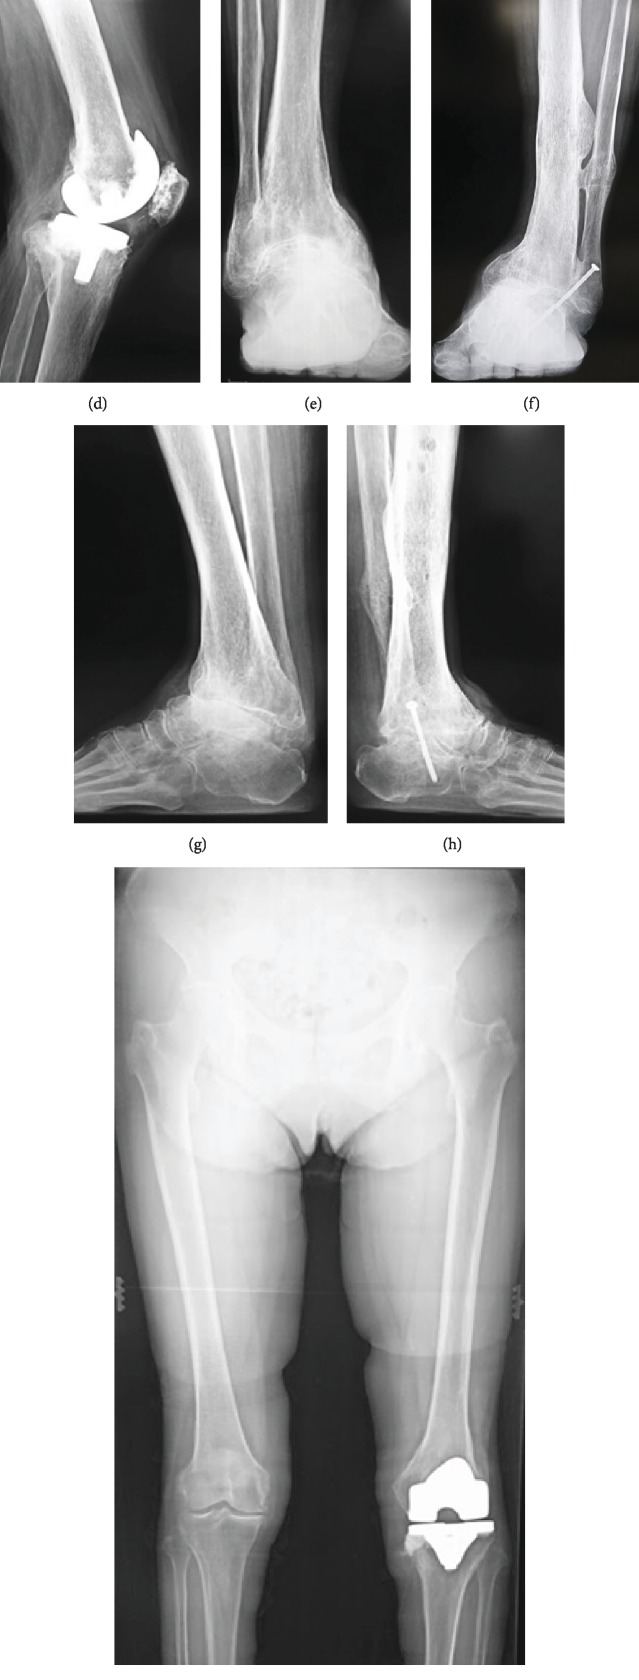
X-rays seven years after surgery. (a–i) It was possible to avoid extreme shortening and to obtain satisfactory alignment with the same leg length as the unaffected side.
